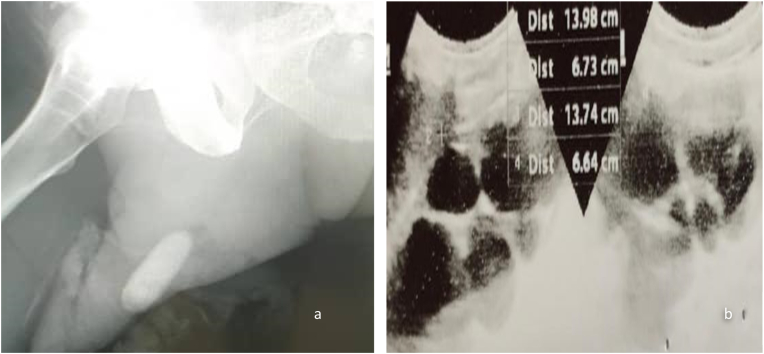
Pelvic X-ray showing penile stone (a); Bilateral Hydronephrosis (b).
